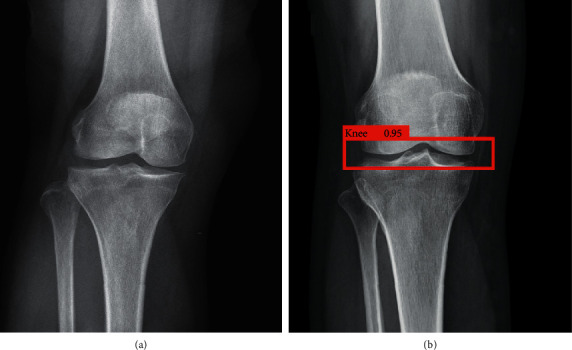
(a) Original radiograph image of the knee joint. (b) Result of the segmentation using YOLO.
